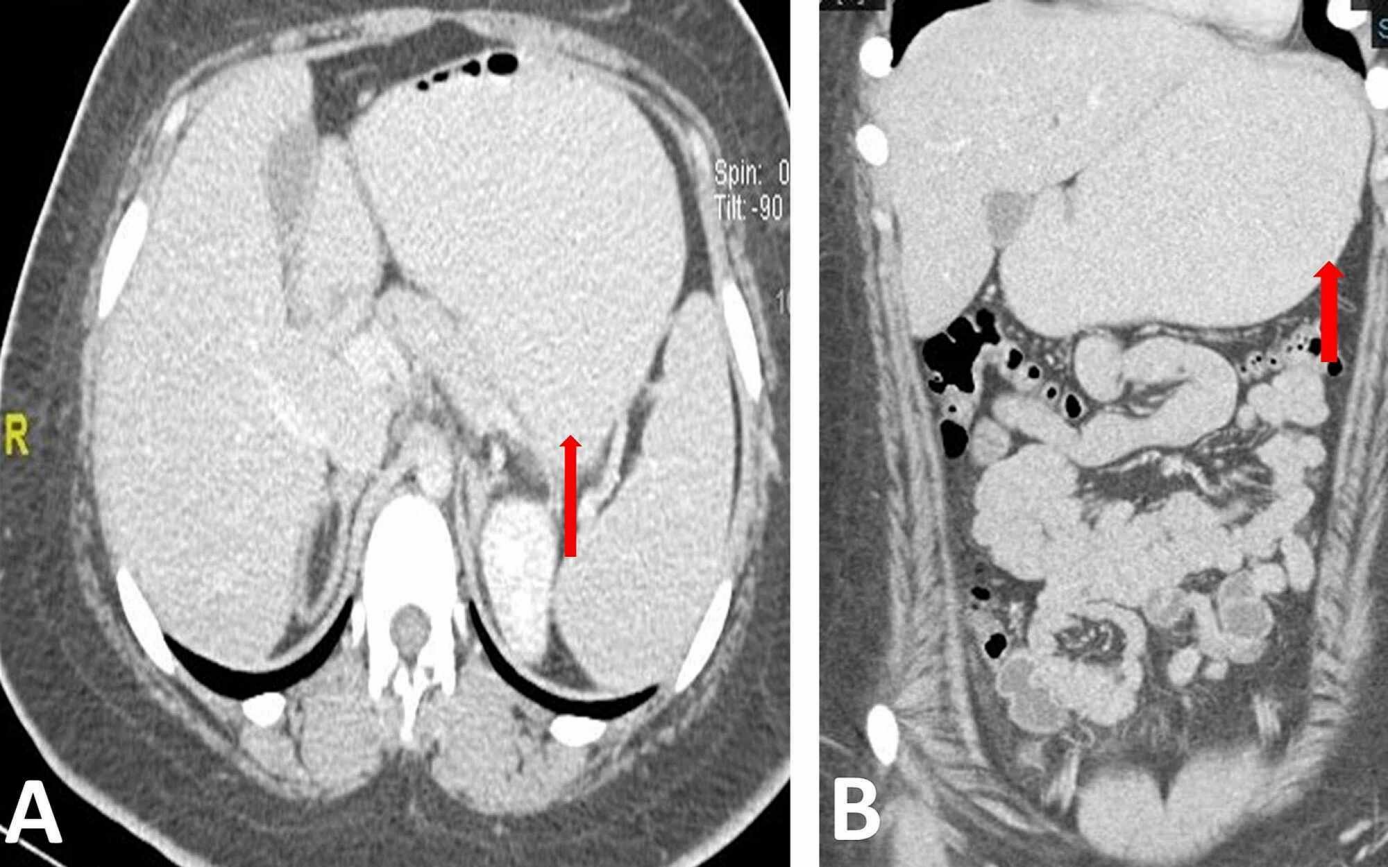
Sagittal (A) and coronal (B) computed tomography scans showing an isolated moderate-grade gastric distension (red arrow), without evidence of distal gastric, pyloric, or proximal duodenal obstructing masses.
